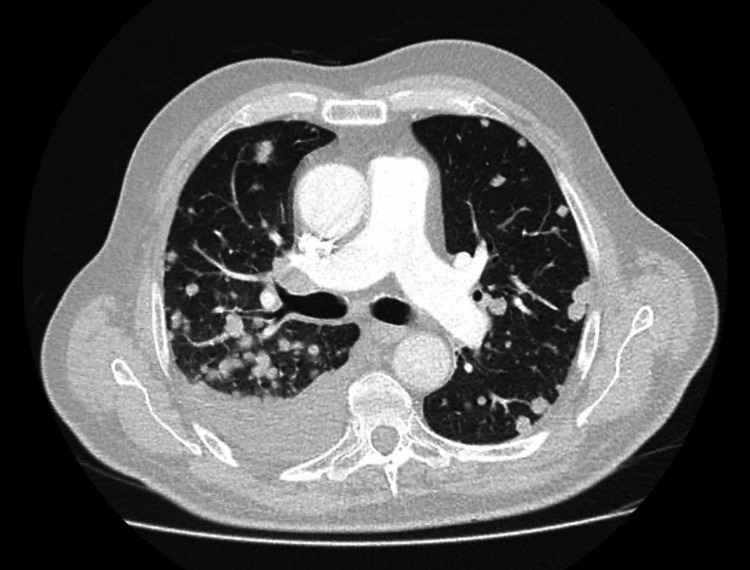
Follow-up chest CT after three cycles on docetaxel and carboplatin (Case 1) Progression of pulmonary metastatic lesions along with de novo pleural effusion.
